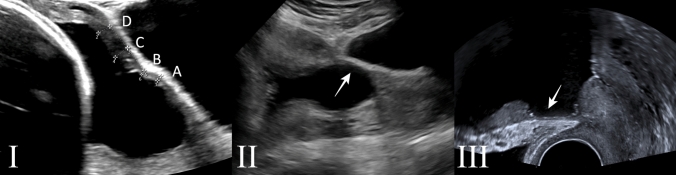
Ultrasound imaging showing: (I) the assessment of the lower uterine segment according to the study protocol at A, B, C, and D. (II) Transabdominal view of a bulging SC scar dehiscence (arrow). (III) Transvaginal view of a CS scar dehiscence with myometrial thinning (arrow)
